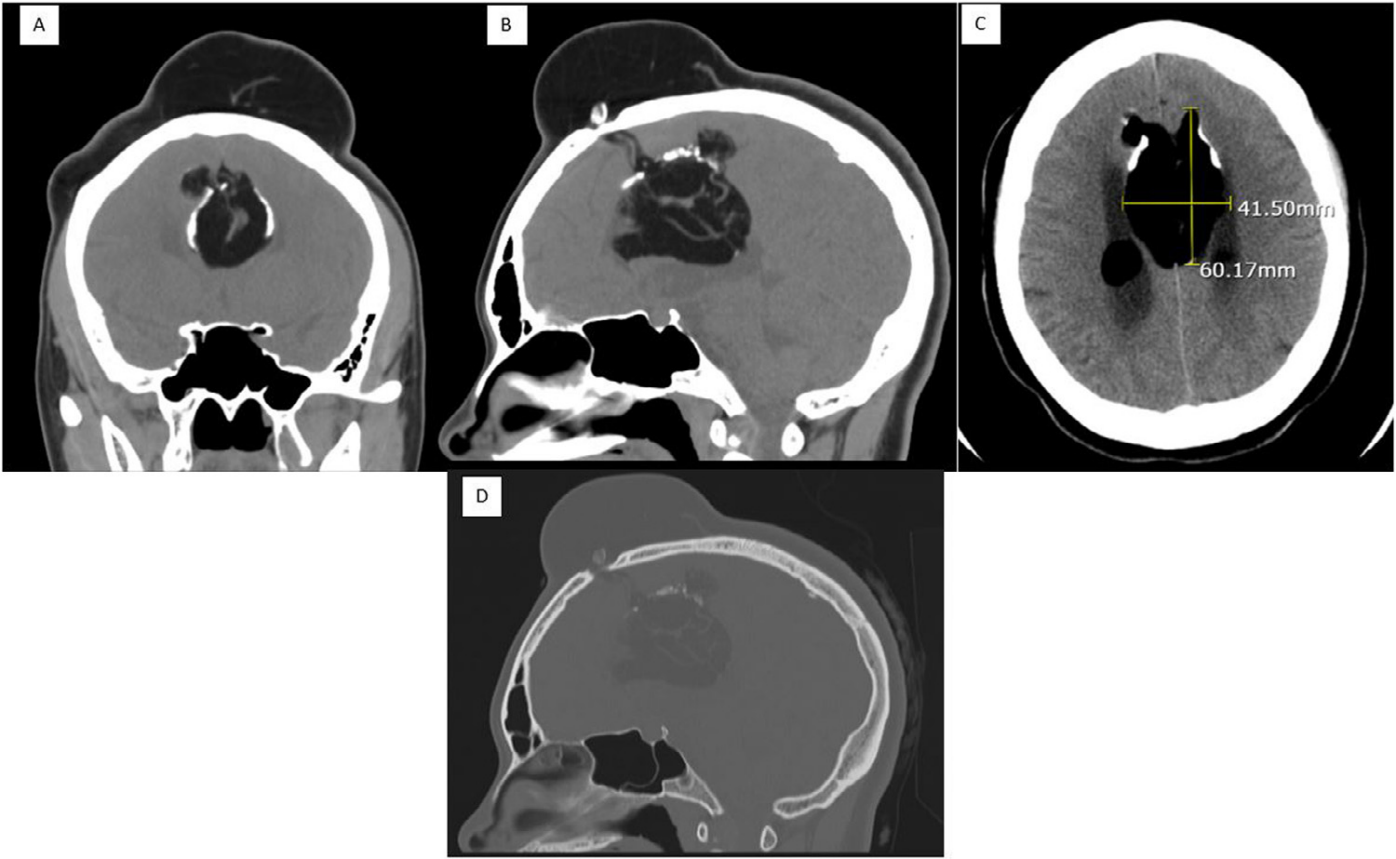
Head CT scan without contrast from May 2021. (A) Coronal view showing the interhemispheric lipoma. (B) Sagittal view showing the intracranial lipoma communicating with the scalp which has enlarged from the prior CT scan to 6 cm by 4.1 cm. (C) Axial view showing the interhemispheric and invasion into the body of the lateral ventricles bilaterally. (D) Sagittal view in bone window showing the intracranial lipoma communicating with the scalp.
